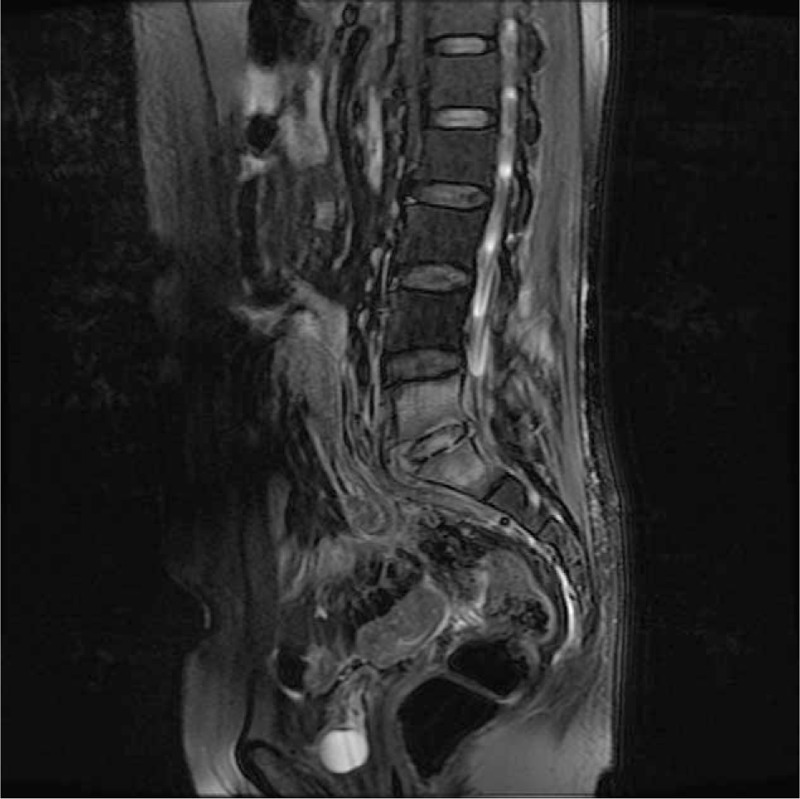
MRI after mesh removal 2 wk later: bony destruction of the lower part of the L5 vertebra and the dome of the sacrum (arrow). MRI = magnetic resonance imaging.
